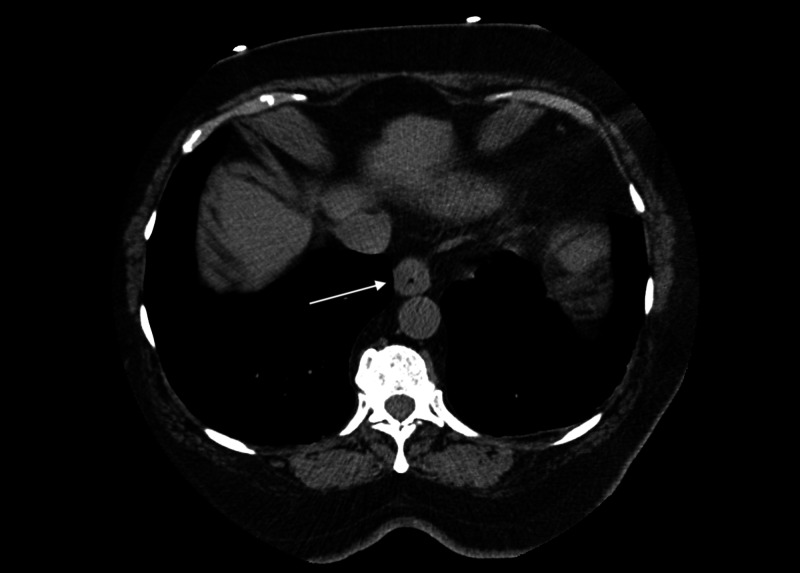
Non-contrasted computed tomography noting circumferential thickening of distal esophagus (arrow)
